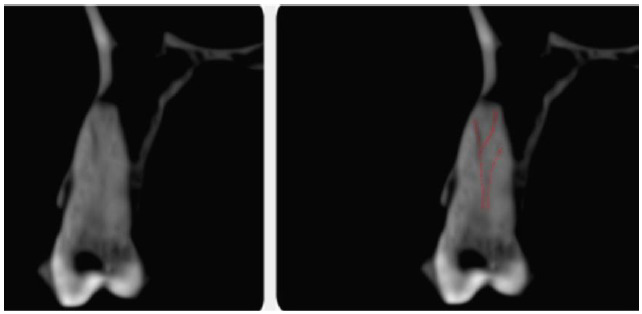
Cone beam computed tomography (CBCT) image of the tooth (coronal view)
